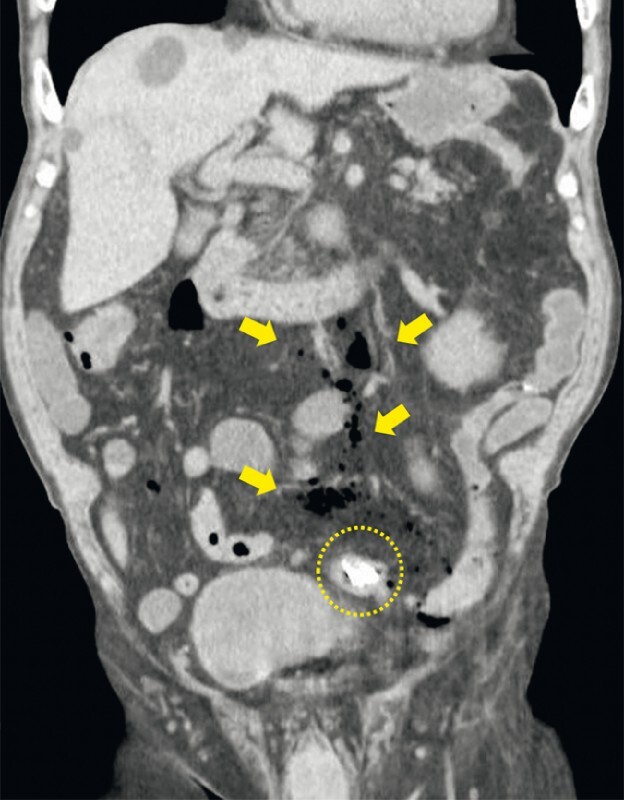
Image from a computed tomography scan performed immediately after the colonoscopy showing the clip used to suture the perforation of the sigmoid colon (dotted yellow line) and air leakage outside of the colon (yellow arrows).
